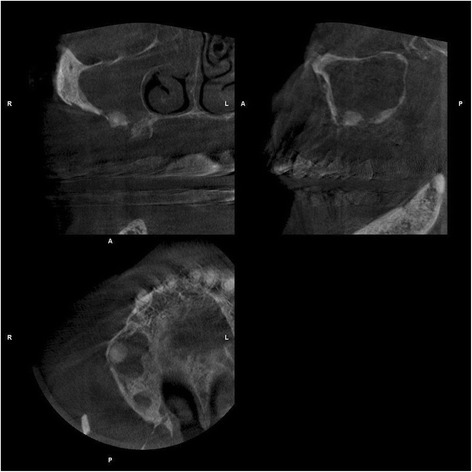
Cone beam CT showing a sequestrum and sinusitis in a patient without intraoral bone exposure
